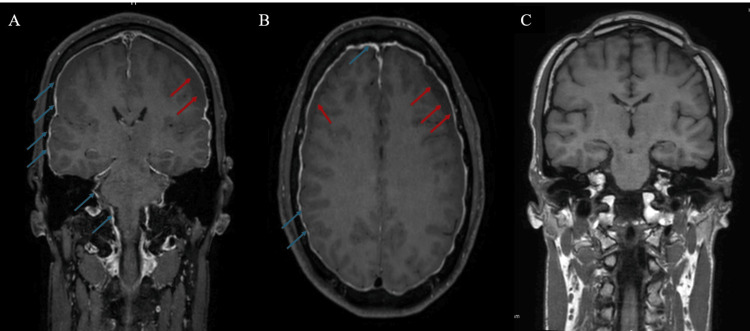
Pre-operative and six months post-operative brain MRI Spontaneous intracranial hypotension. (A) Coronal and (B) axial pre-operative T1-weighted gadolinium-enhanced MRI demonstrate diffuse pachymeningeal enhancement (thin blue arrows), brainstem sagging, and bilateral subdural collections (thin red arrows). (C) Post-operative T1-weighted gadolinium-enhanced coronal MRI shows a complete resolution of pachymeningeal enhancement and bilateral subdural collections. MRI: magnetic resonance imaging
